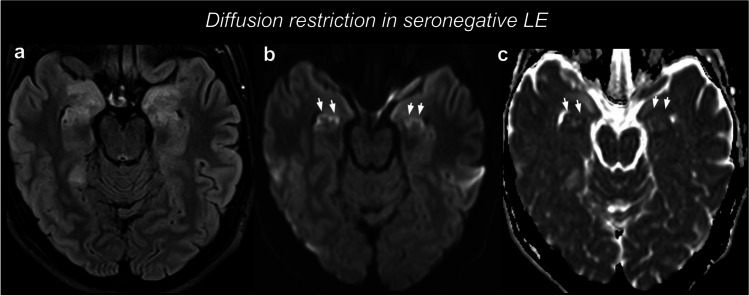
Limbic encephalitis with diffusion restriction. In this seronegative case, T2-weighted FLAIR images (a) showed a marked symmetric swelling and hyperintensity of the amygdala and head of the hippocampus. DWI (b) and ADC (c) revealed a gyriform diffusion restriction in the hippocampus (arrows), with ADC values as low as 0.58 × 10^3^ mm^2^/s (right) and 0.72 × 10^3^ mm^2^/s (left). After a diagnostic work-up, a diagnosis of seronegative AE was posed
